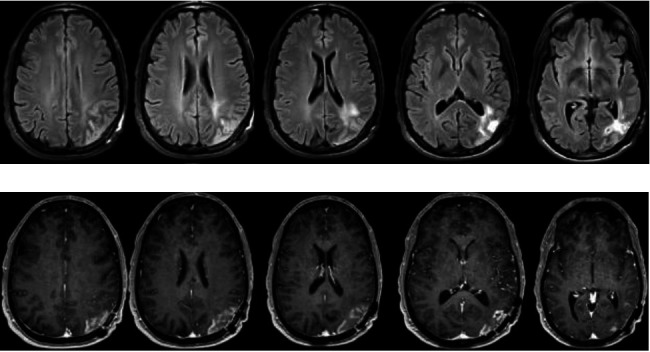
Brain magnetic resonance imaging with and without contrast. Brain MRI: (a) T2 FLAIR showing left parieto-occipital resection cavity with surrounding T2 FLAIR hyperintensity. (b) T1 postcontrast showing nonspecific faint rim of peripheral ribbon like enhancement.
